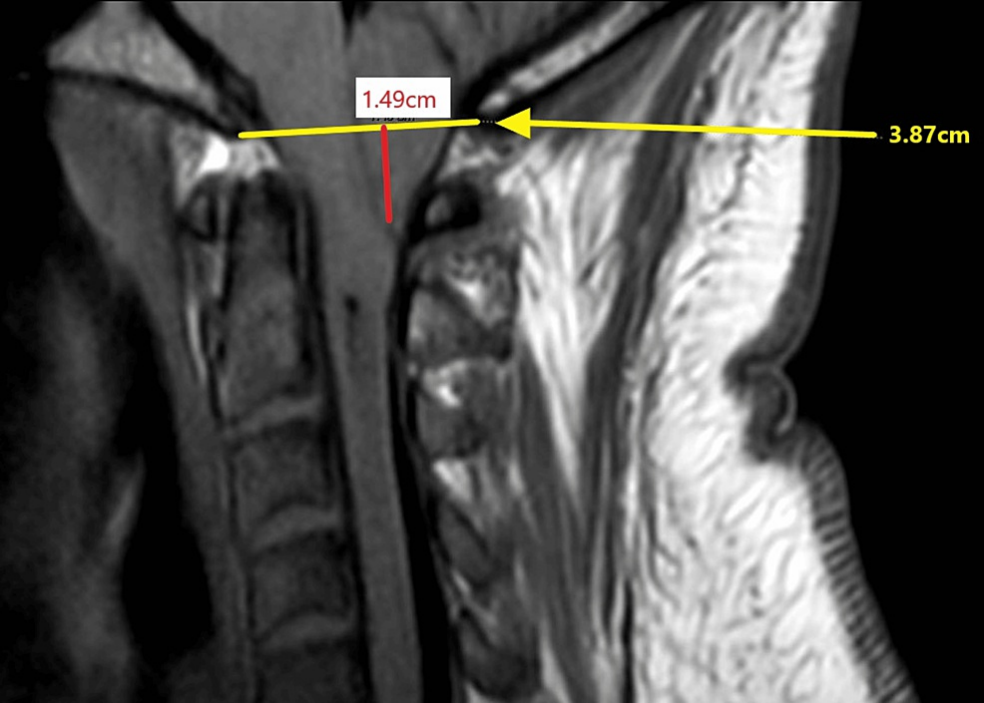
MRI cervical spine (sagittal view) demonstrating cerebellar ectopia Red line: Cerebellar ectopia extending 1.5 cm below the foramen magnum. Yellow line: Representing the level of the foramen magnum (3.87 cm in width)
